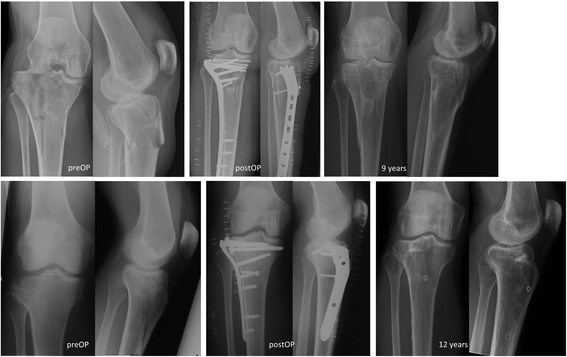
Radiographs showing preoperative, postoperative, and long-term condition after tibial head fracture. Patient 1 nine years after bicondylar tibial head dislocation fracture. Patient 2 sustained a lateral depression type tibial head fracture 12 years ago
